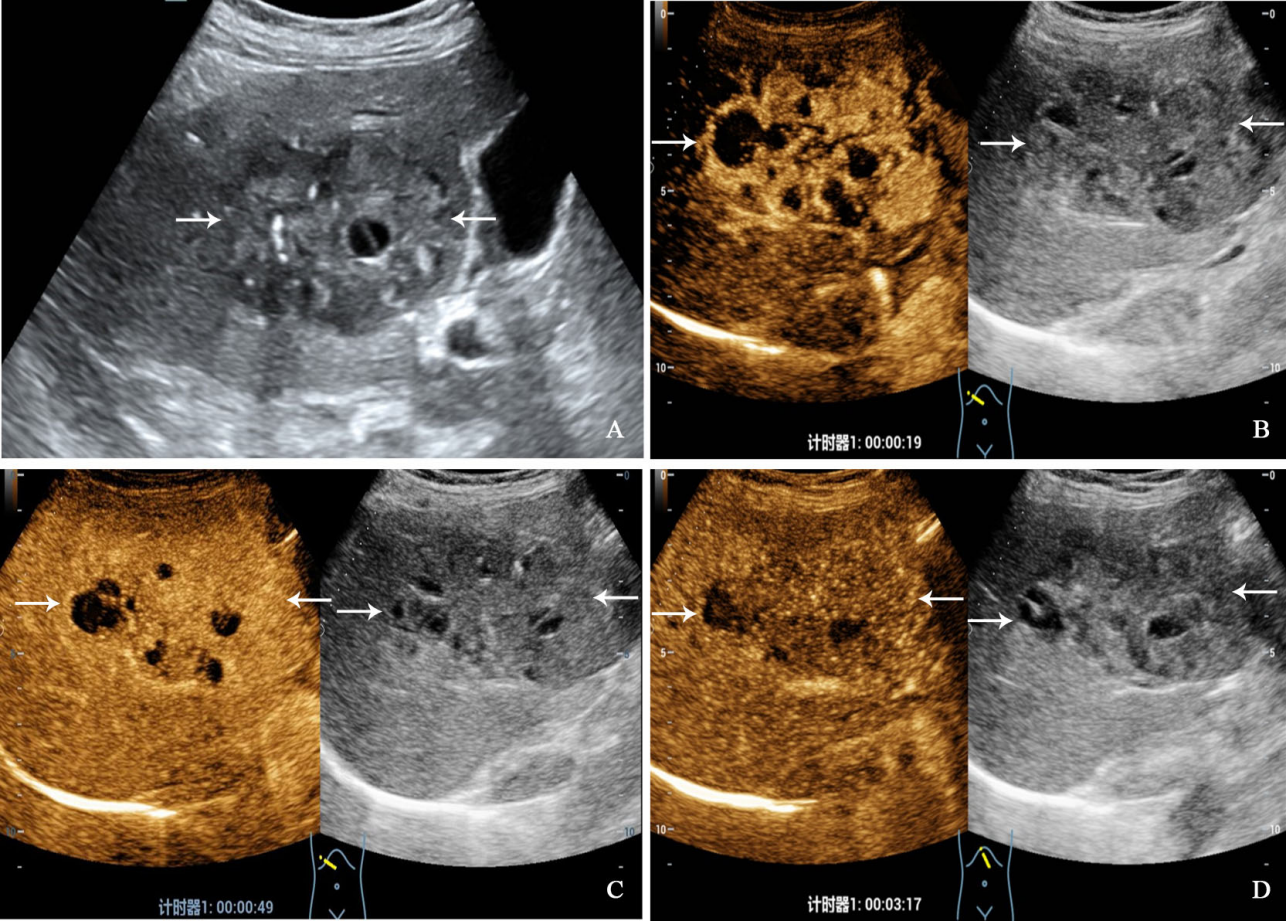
A 68-year-old woman with primary neuroendocrine tumor. The patient had no underlying liver disease. Conventional ultrasound showed that a mixed echoic tumor with focal calcification and largest diameter of 10.0 centimeters in anterior segment of the liver (A). In the arterial phase of contrast-enhanced ultrasound, the tumor showed heterogeneous hyperenhancement (B), and began washout before 60 seconds (C). hypoenhancement in the late phase (D).
